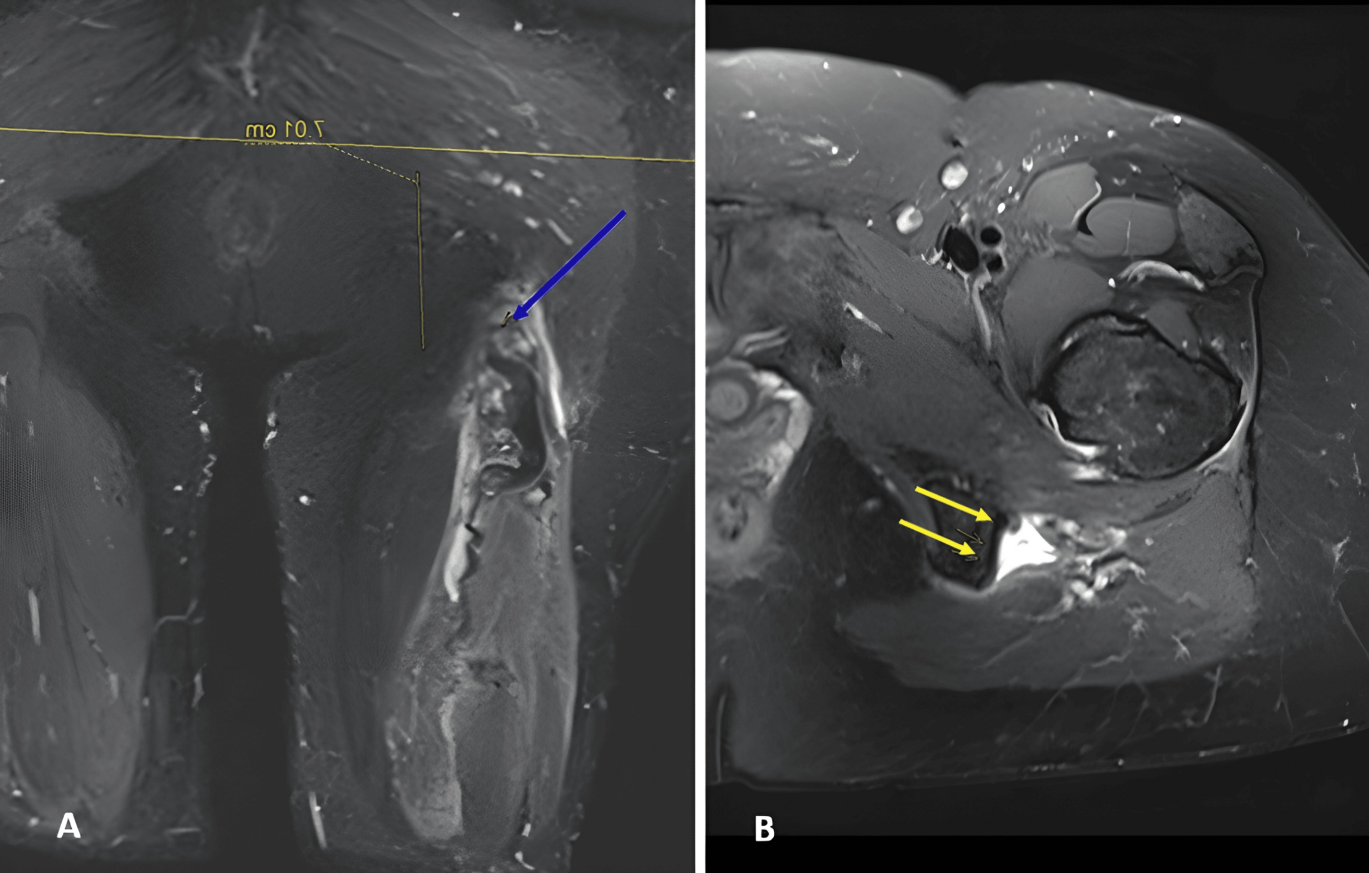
Preoperative MRI (A) Preoperative coronal STIR magnetic resonance image showing the retracted tendon stump and its distance from the ischial tuberosity (blue arrow); (B) Preoperative axial T2 fat-suppressed image demonstrating complete avulsion of the hamstring tendons (two yellow arrows). STIR: Short Tau Inversion Recovery Image Source: Faculty of Medical Science and Health, Pontifical Catholic University of São Paulo; Used with Permission
